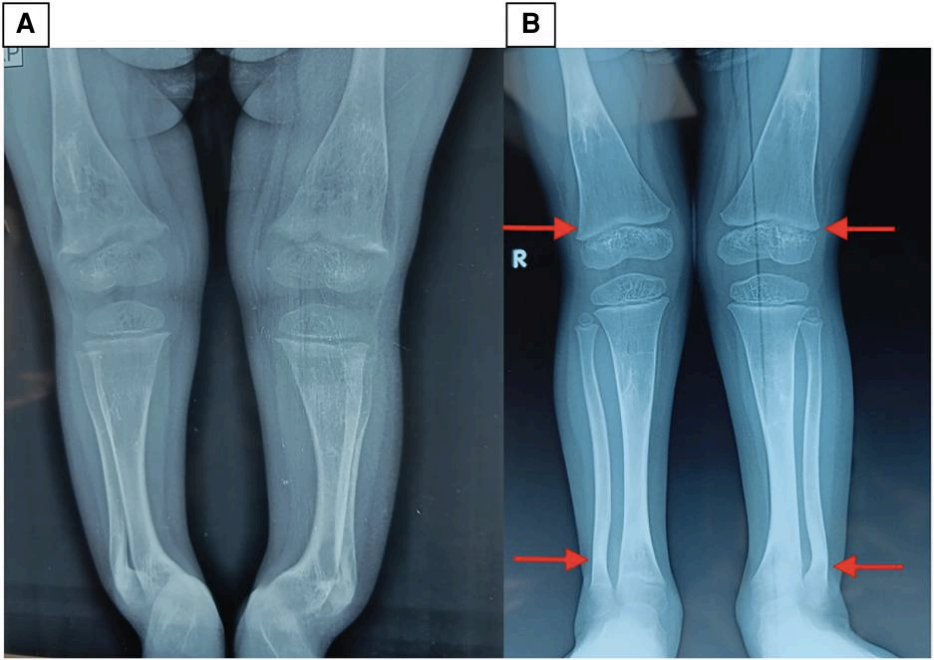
X-rays of the proband before (A) and after (B) treatment. X-ray of bilateral lower limbs of the proband before (A) and after (B) treatment with cholecalciferol. Panel A shows metaphyseal fraying, splaying, and cupping of the lower femoral metaphysis and anterolateral bowing in bilateral lower tibia and fibula. Panel B shows resolution of the metaphyseal changes and improvement in deformity in the tibia and fibula (arrows).
